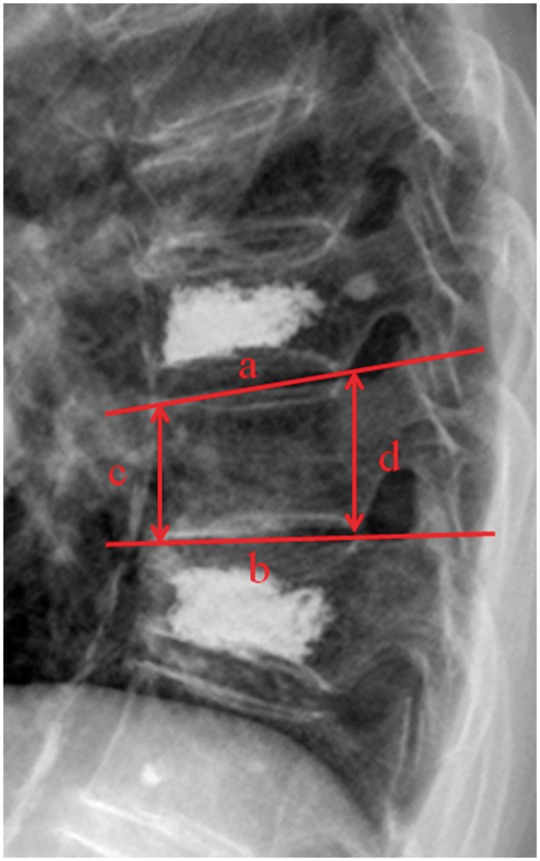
Lateral radiograph showed that the sandwich vertebral body located between two cement treated vertebrae. Cobb angle was the angle between a and b. The ratio between anterior height and posterior height was c/d
